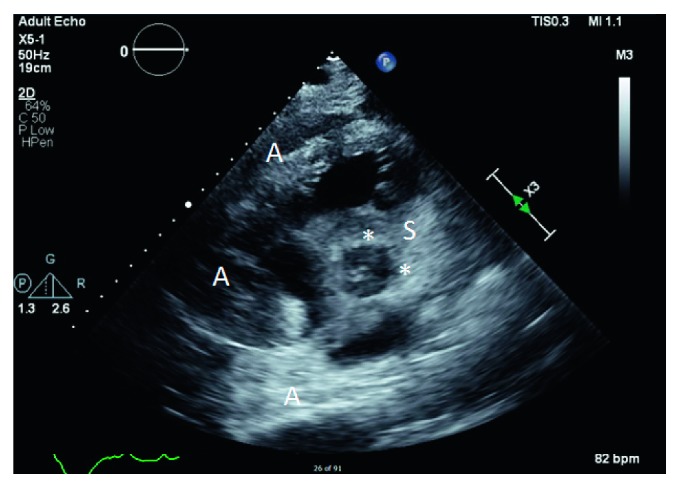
Transthoracic echo—parasternal short view. There is extensive homogenous echodense mass superiorly (S) and anteriorly (A) in relation to the cardiac tissue. The most striking feature is that despite the infiltration and surrounding of the cardiac structures of the right atrium, right ventricle, and the aortic valve, there is no evidence of tamponade physiology. The homogenous echodensity encases the ascending aorta at the level of the aortic valve (^∗^), masking the coronary ostia leading into the coronary arteries.
